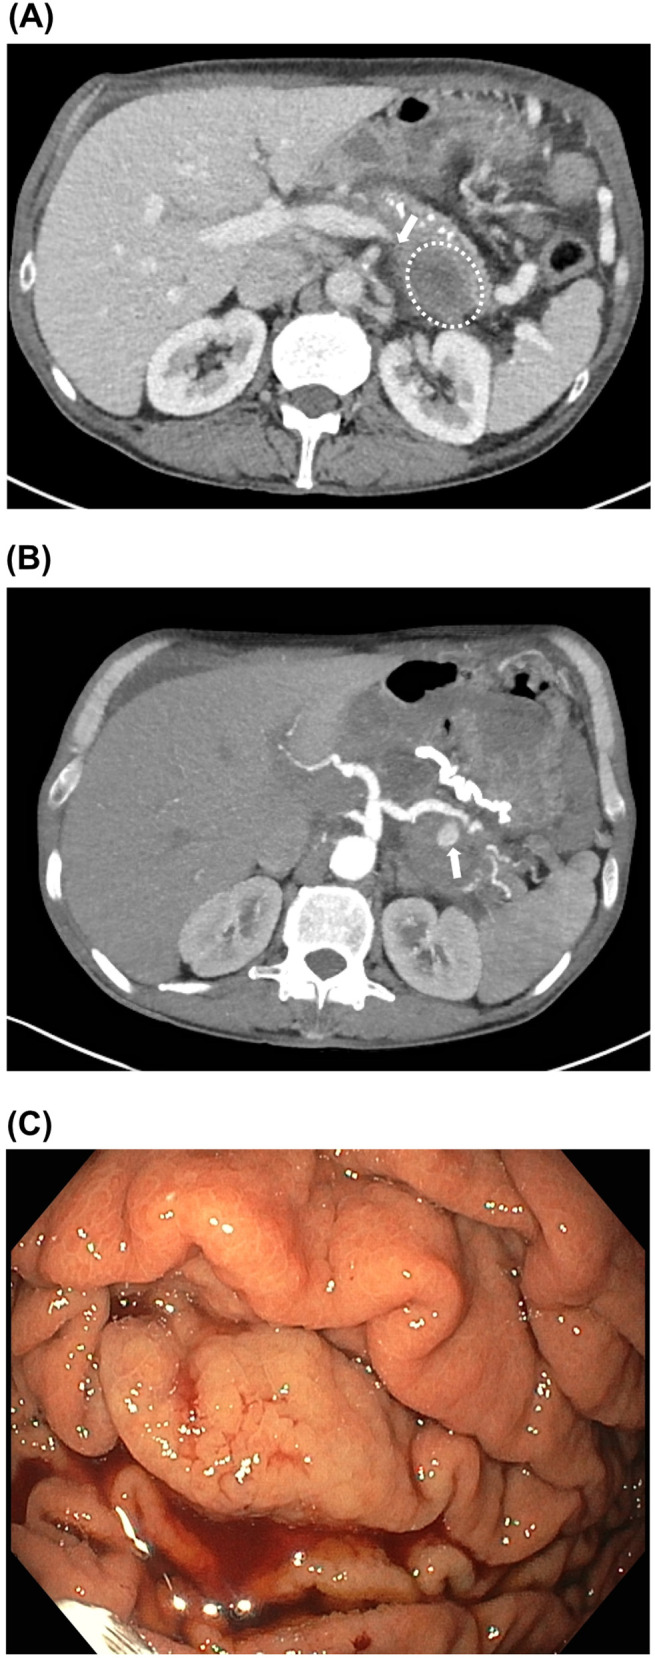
Pancreatitis with complications and portal hypertensive gastropathy. (A) CT scan performed in July 2017 showing severe flare of chronic pancreatitis with presumed thrombosis/compression of the *V. lienalis* (white arrow) and a walled-off necrosis (dotted circle). (B) CT scan from July 2017 showing the pseudoaneurysm of the *V. lienalis* as a complication of the severe pancreatitis. (C) Gastroscopy in August 2024 showing signs of portal hypertensive gastropathy with minimal fresh bleeding.
